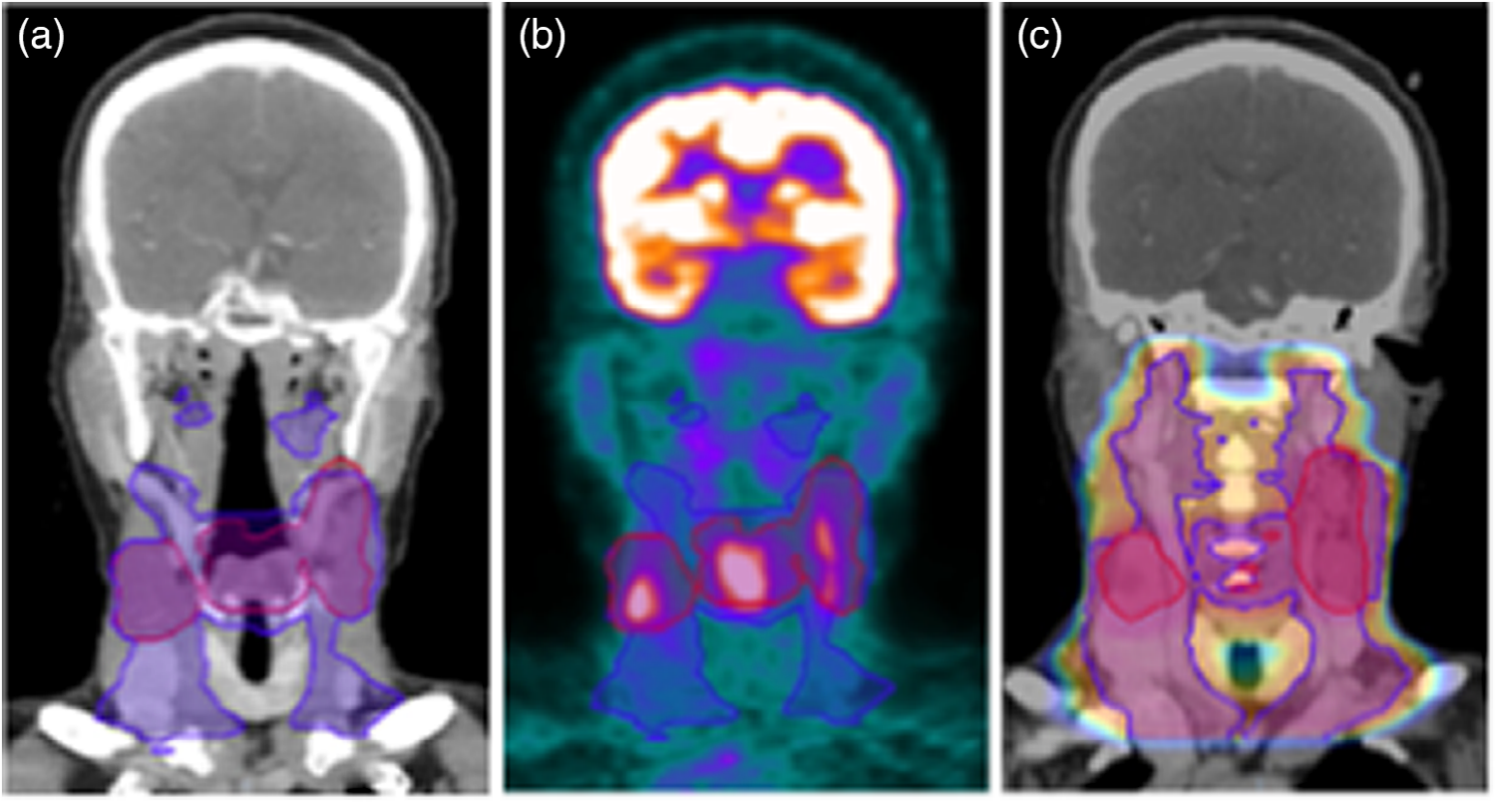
Imaging of a Stage T3N3b squamous cell carcinoma of the glottic larynx in a 70‐year‐old female procured from our institution. (a) Coronal view of the planning CT with clinical target volumes (CTV 7000 and CTV 6300) outlined. (b) Coronal view of the PET scan showing CTV 7000 and CTV 6300, with a window level of 0 to 8 standard uptake value (SUV). (c) Coronal view of the planning CT image with CTV 7000 and CTV 6300 contours, augmented with a dose color wash: dose window level set from 28 to 70 Gy.
